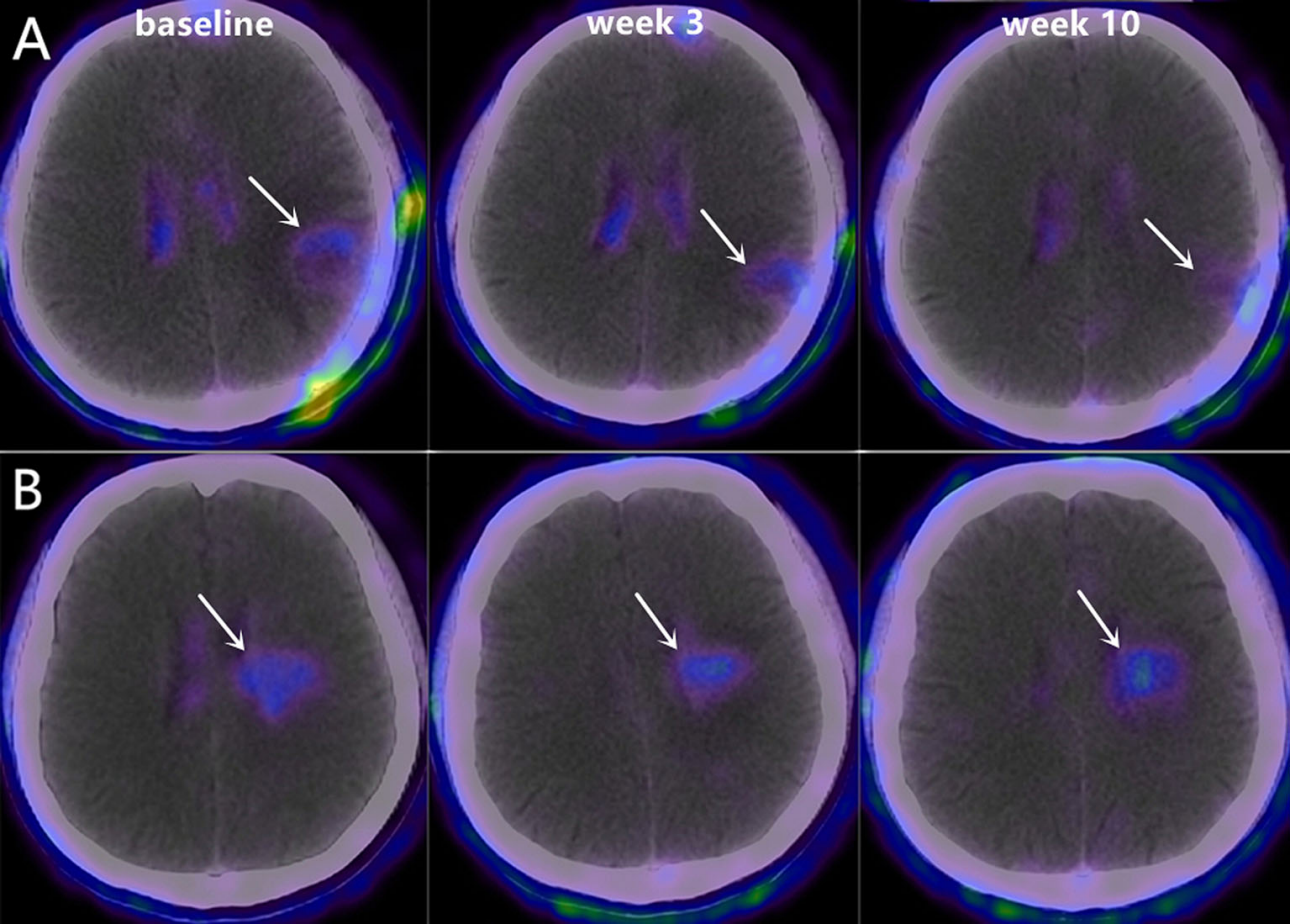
Representative ^18^F-RGD PET/CT scans at baseline, week 3 and week 10 in two patients with PFS of 9.65 months (A, Male, 48 years old, decrease in SUV_mean_=-0.45) and 7.45 months (B, Male, 62 years old, decrease in SUV_mean_=0.25).
